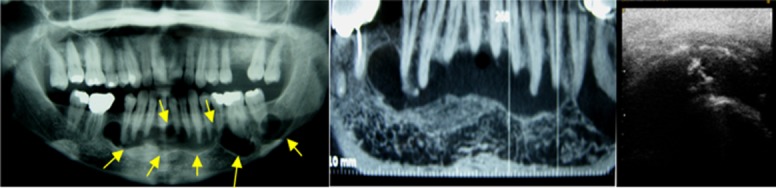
A case of simple bone cyst. (A) Panoramic view reveals extensive radiolucent lesion with scalloped border in left mandible. (B) CBCT shows large lytic lesion in left mandible. (C) Ultrasound image shows irregular hyperechoic pattern presenting air inside the lesion.
